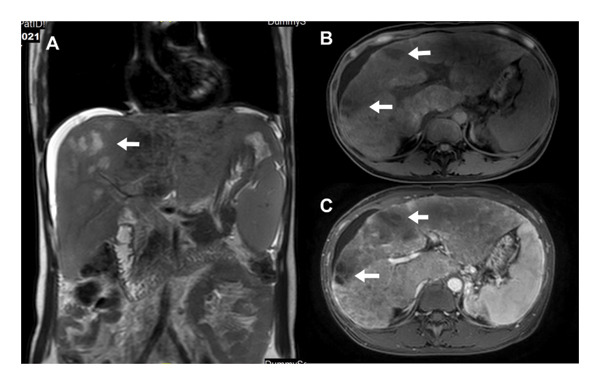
MRI image of Case 2: (A) coronal T2‐weighted image displaying a diffusely inhomogeneous signal in the liver parenchyma, visible ascites surrounding the liver, and multiple patchy high‐signal shadows within the mass in the right anterior lobe. (B) Axial T1‐weighted image showing low‐signal mass shadows in the left medial and right anterior lobes of the liver. (C) Axial‐enhanced scan during the portal venous phase indicating mass‐like reduced enhancement of tumor shadows).
